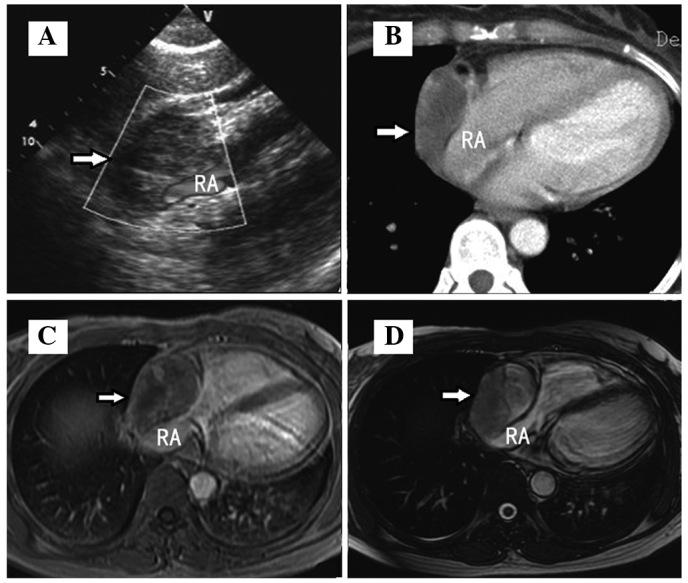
Echocardiography and radiological images of pericardial synovial sarcoma. (A) Echocardiography revealed a mass (arrow) within the pericardial space predominantly over the right atrium. (B) Contrast-enhanced computed tomography (CT) revealed a low-attenuation lesion (arrow) in the pericardium, with inhomogeneous peripheral enhancement. (C) T1-weighted post-gadolinium magnetic resonance imaging (MRI) identified mild heterogeneous enhancement (arrow). (D) 2D FIESTA sequence images revealed a 3.4×5.2-cm, high-signal, heterogeneous multilocular mass (arrow). FIESTA, fast imaging employing steady-state acquisition; RA, right atrium.
